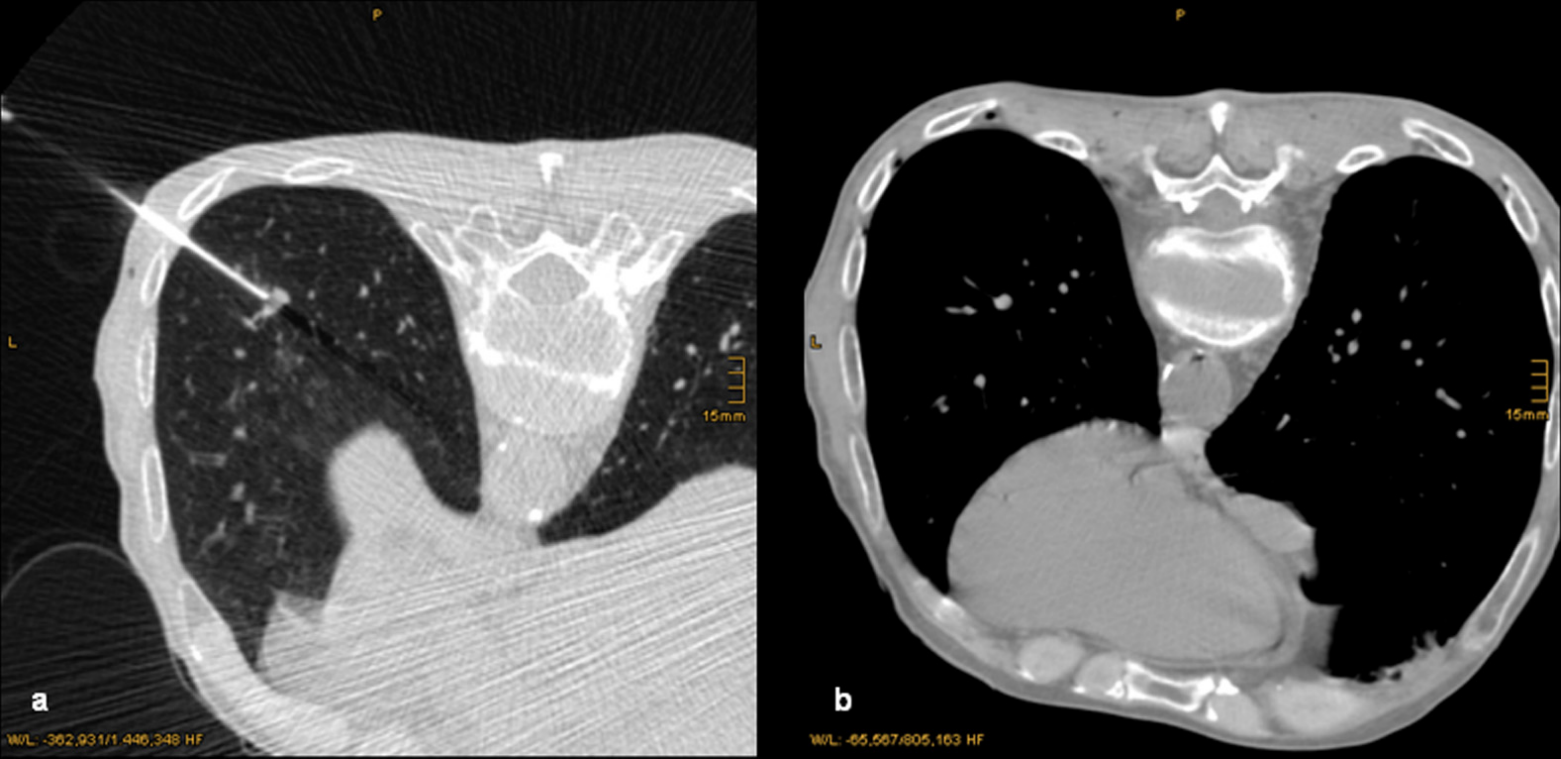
a and b: Transversal CT-slice of a 48 y/o patient during the biopsy of a small nodule located in the left lower lobe (a). The CT-Scan after biopsy showed a SAE in the left atrium, the aorta, and intercostal arteries (b). The patient suffered from a transient amaurosis.
